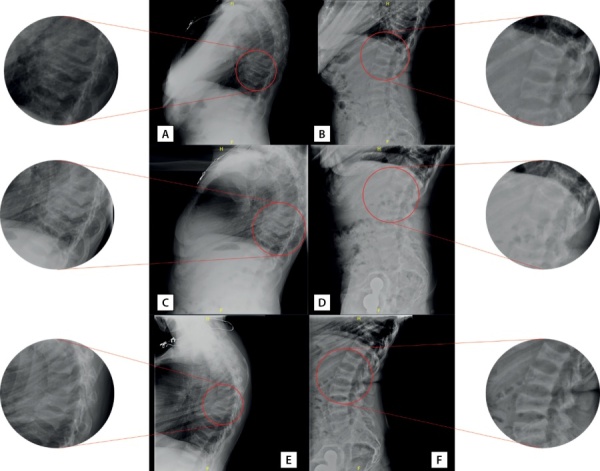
Рисунок 2. Рентгенография позвоночника пациентки А.: грудной (А, C, E) и поясничный (В, D, F) отделы в боковых проекциях. А и В — июль 2018 г., C и D — сентябрь 2019 г., Е и F — сентябрь 2020 г. Пояснения в тексте.Figure 2. X-ray of patient A’s spine: thoracic (A, C, E) and lumbar (B, D, F) sections in lateral views. A and B — July 2018, C and D — September 2019, E and F — September 2020. Explanations in the text.
